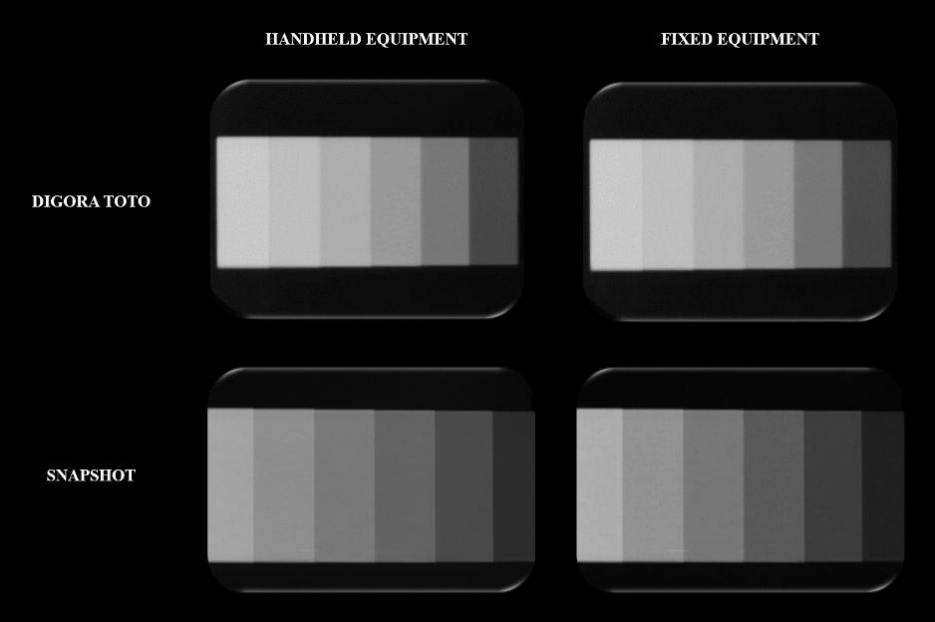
Radiographs of an aluminum step-wedge obtained with handheld portable X-ray and fixed intraoral X-ray equipment using the Digora Toto and Snapshot systems.
